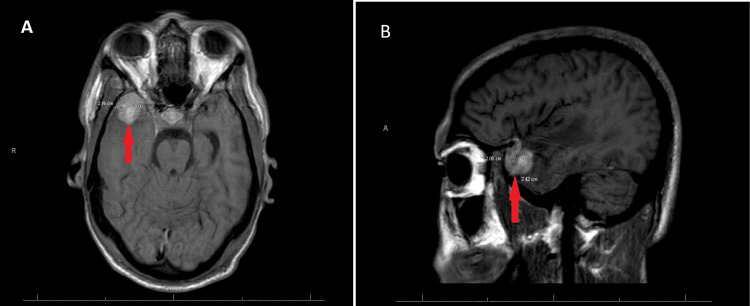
MRI of the brain in coronal and sagittal views (Panels A and B, respectively), with red arrows indicating the lesion at the right anterior temporal pole MRI: magnetic resonance imaging
